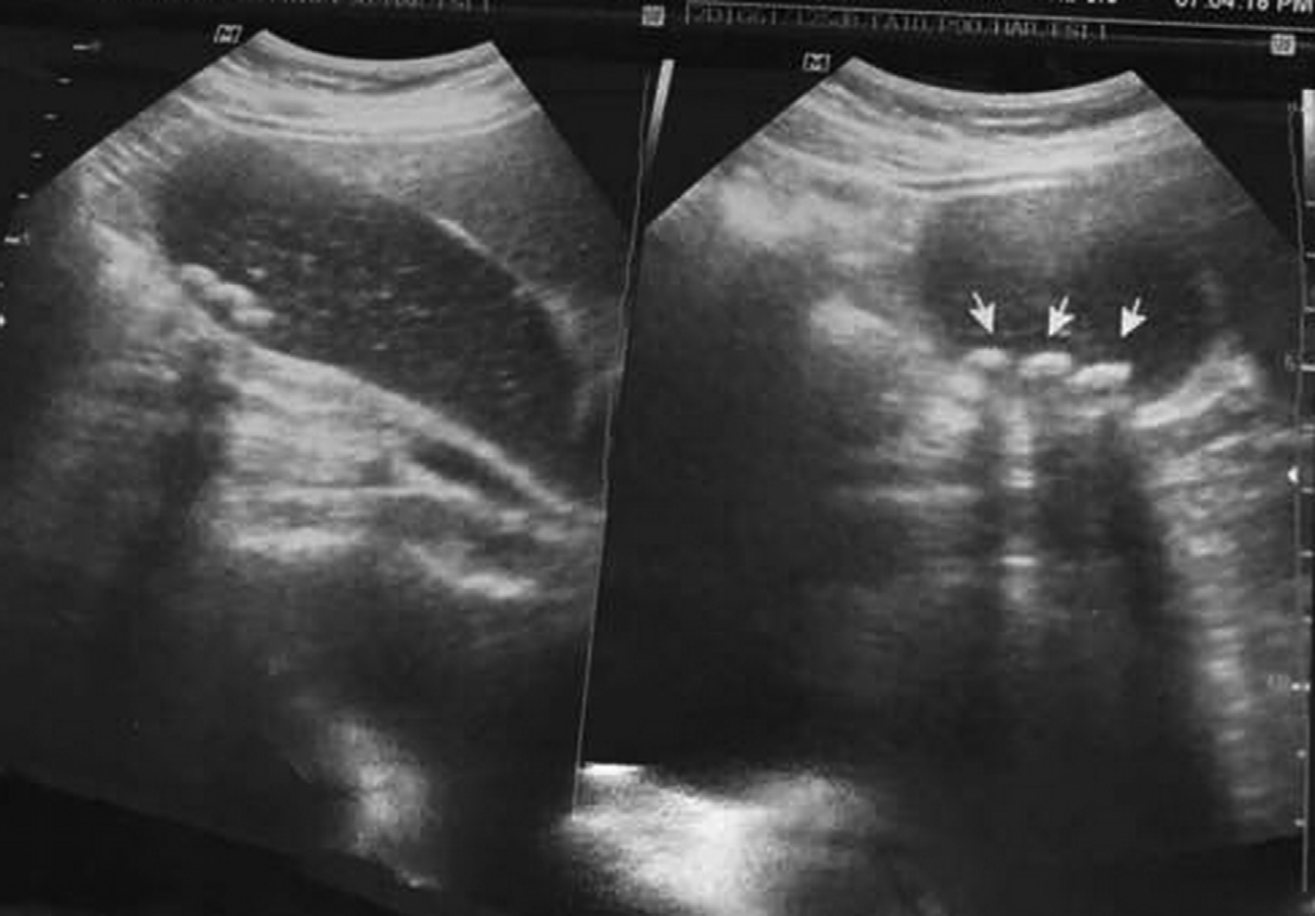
A sonographic picture showing the gall bladder containing multiple stones with no signs of acute inflammation.
